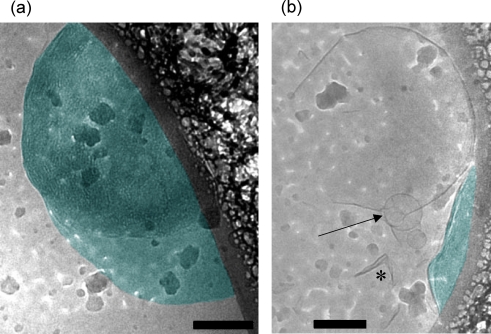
Cryo-TEM images of ME-based aqueous dispersion containing 1.5 wt% lipid, and 0.035 wt% F127 after vitrification from 65°C. The dispersion was ultrasonicated and extruded through 200 nm filters before the investigation. (A) A stabilized ME-based particle with inner cubic structure highlighted in dark cyan. The inner nano-tubular network is clearly visible. Judging from the X-ray data the inner lattice should be of the symmetry Im3m. The overall shape, however is not well defined as compared to other cubosomes known from literature [9], [29], [73]. (B) An adhered cubosome of lengthy shape is seen (dark cyan). In other areas relatively large open vesicles with a cluster of small vesicles is visible (marked by an arrow).
